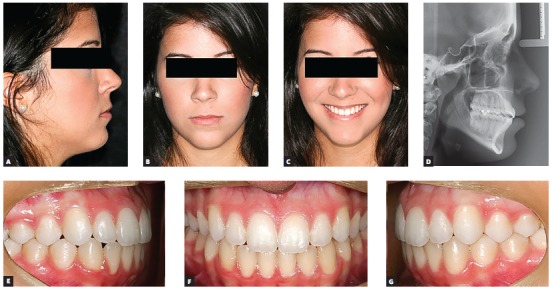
Final photographs: extraoral (A, B, C), radiograph (D) and intraoral (E, F, G).
